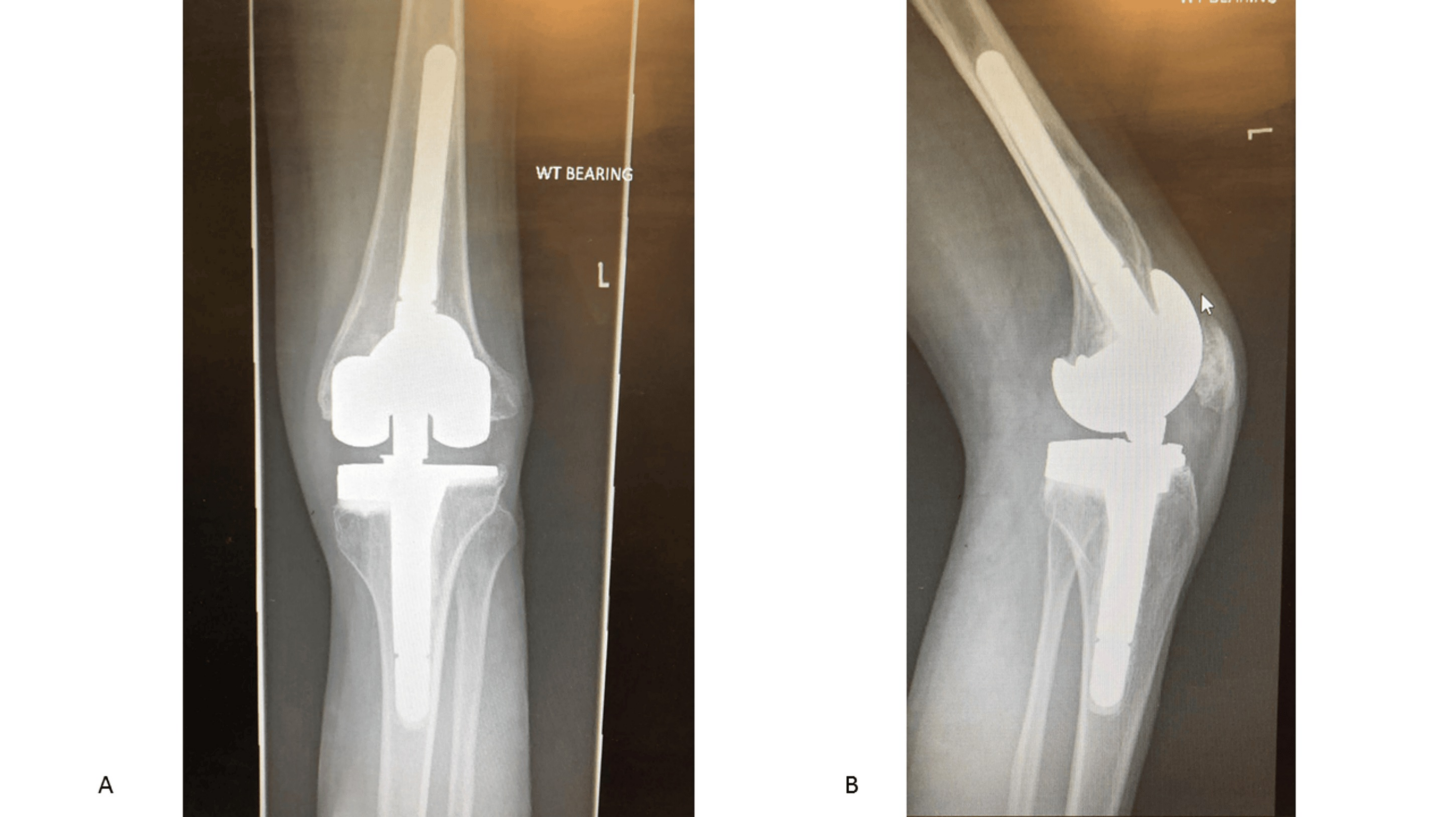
(A) Postoperative anteroposterior X-ray of the left knee at two years and seven months. (B) Postoperative lateral X-ray of the left knee at two years and seven months.
